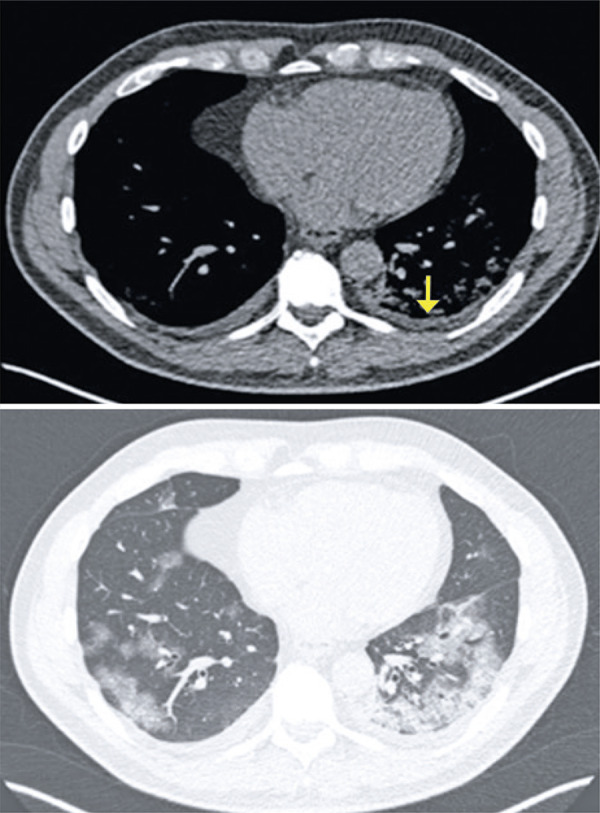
A 63-year-old patient with COVID-19, presenting fever and myalgia seven days. Computed tomography shows bilateral small-volume pleural effusion, ground-glass opacities in the lower lobes and consolidation in the left lower lobe
